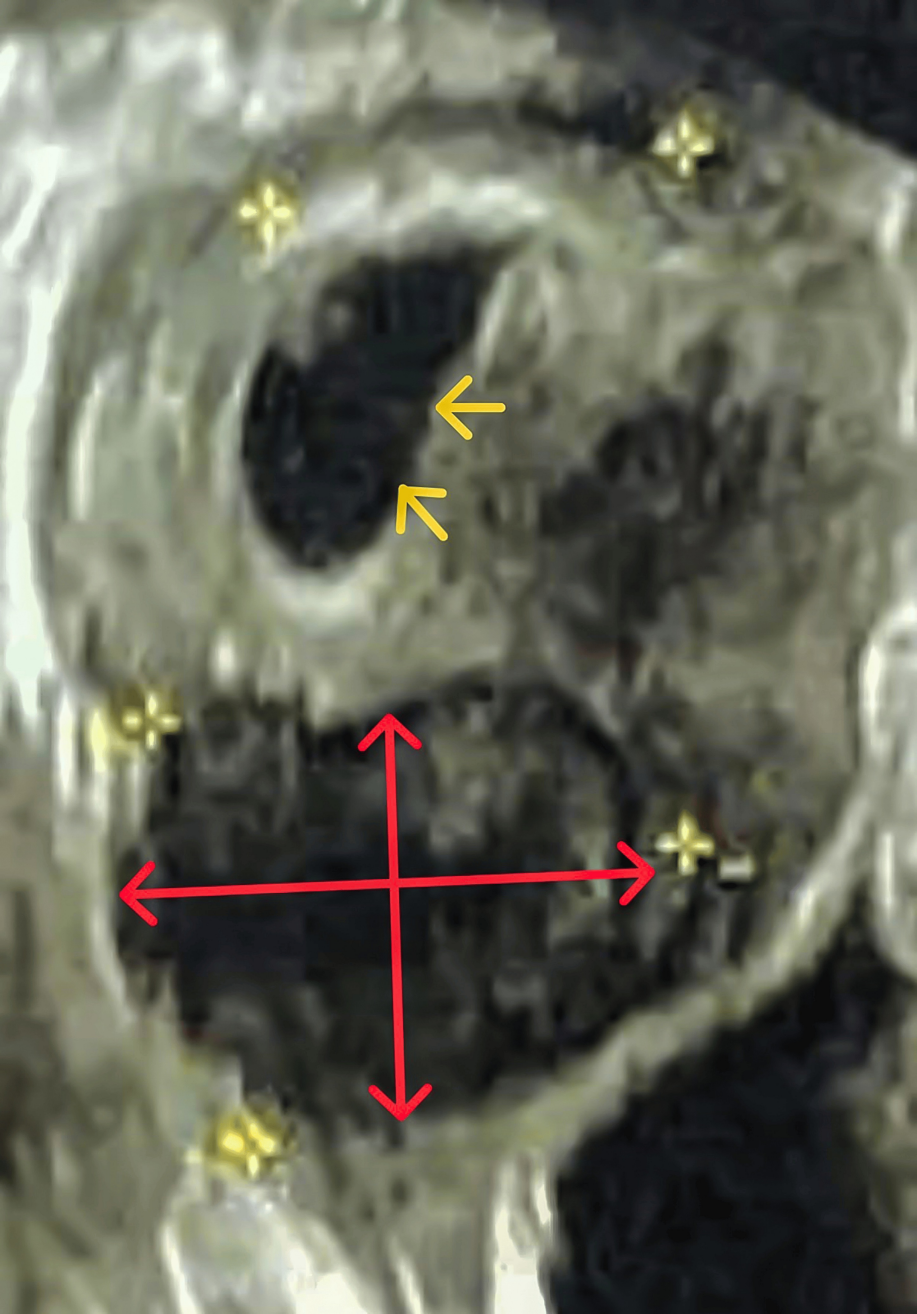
On transvaginal ultrasonography (TVS) a mass was seen in the vagina measuring 5.8x4.2 cm (marked in red). The uterus was normal in size and echo pattern with mild endometrial collection (marked by yellow arrows).
